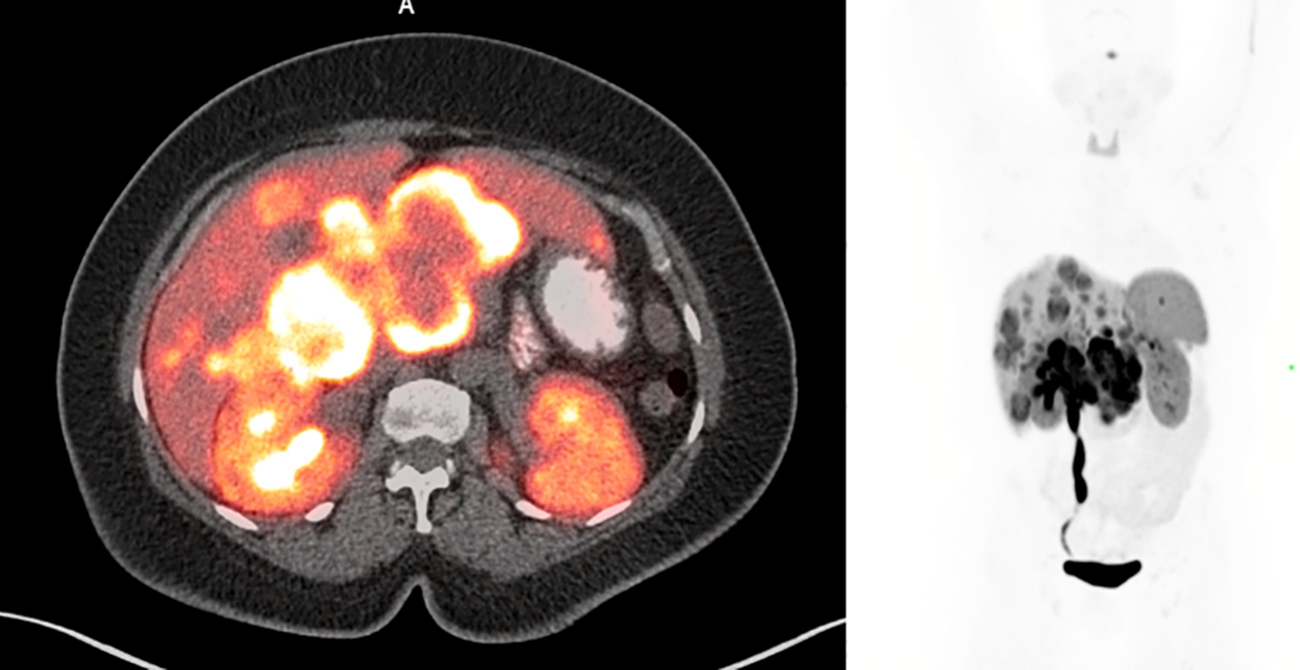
Pre-treatment ^68^Ga-DOTATATE PET/CT showing multiple tracer avid liver lesions and a peripancreatic mass. Peripancreatic mass SUVmax was 36.7.
